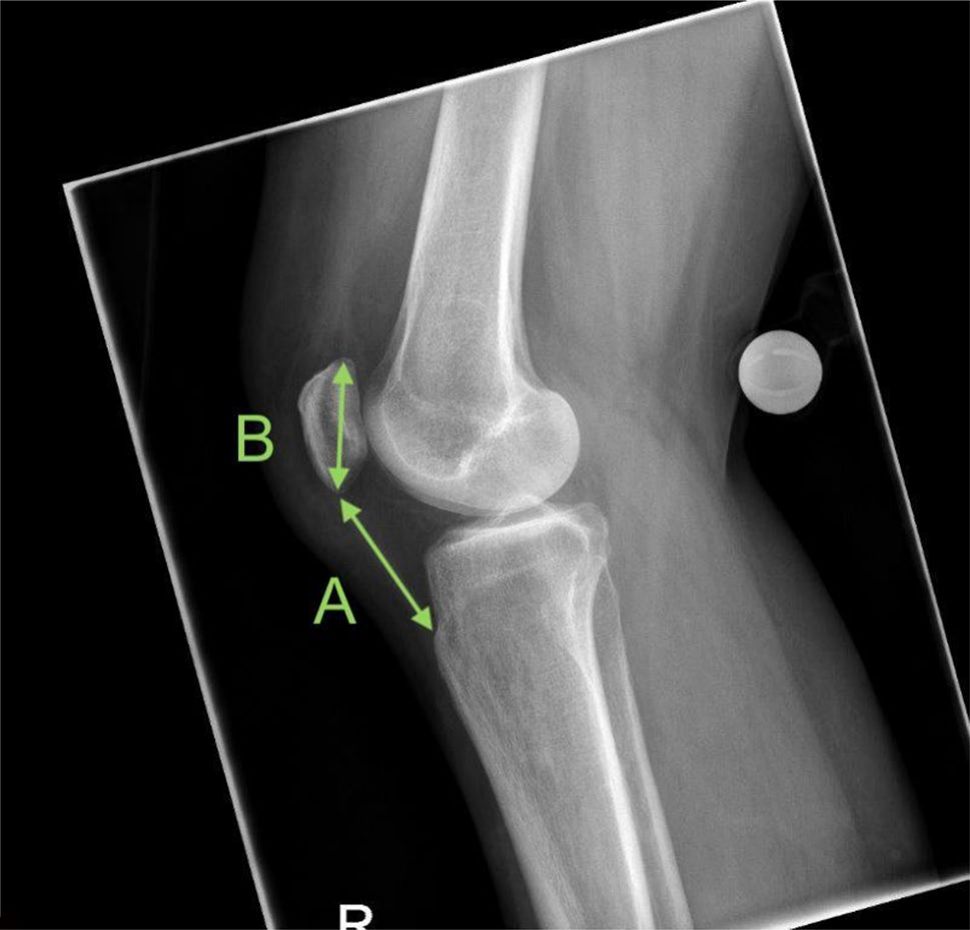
Lateral view (X-ray) of the knee showing the assessment of the Insall–Salvati Ratio (ISR, A/B)
